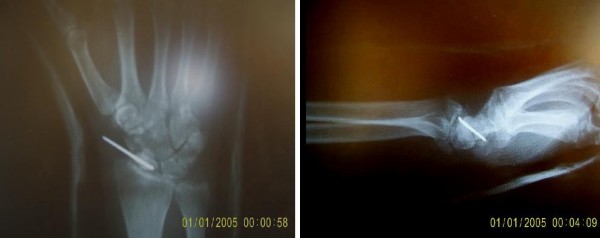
Postoperative AP and lateral radiograph of the wrist.
